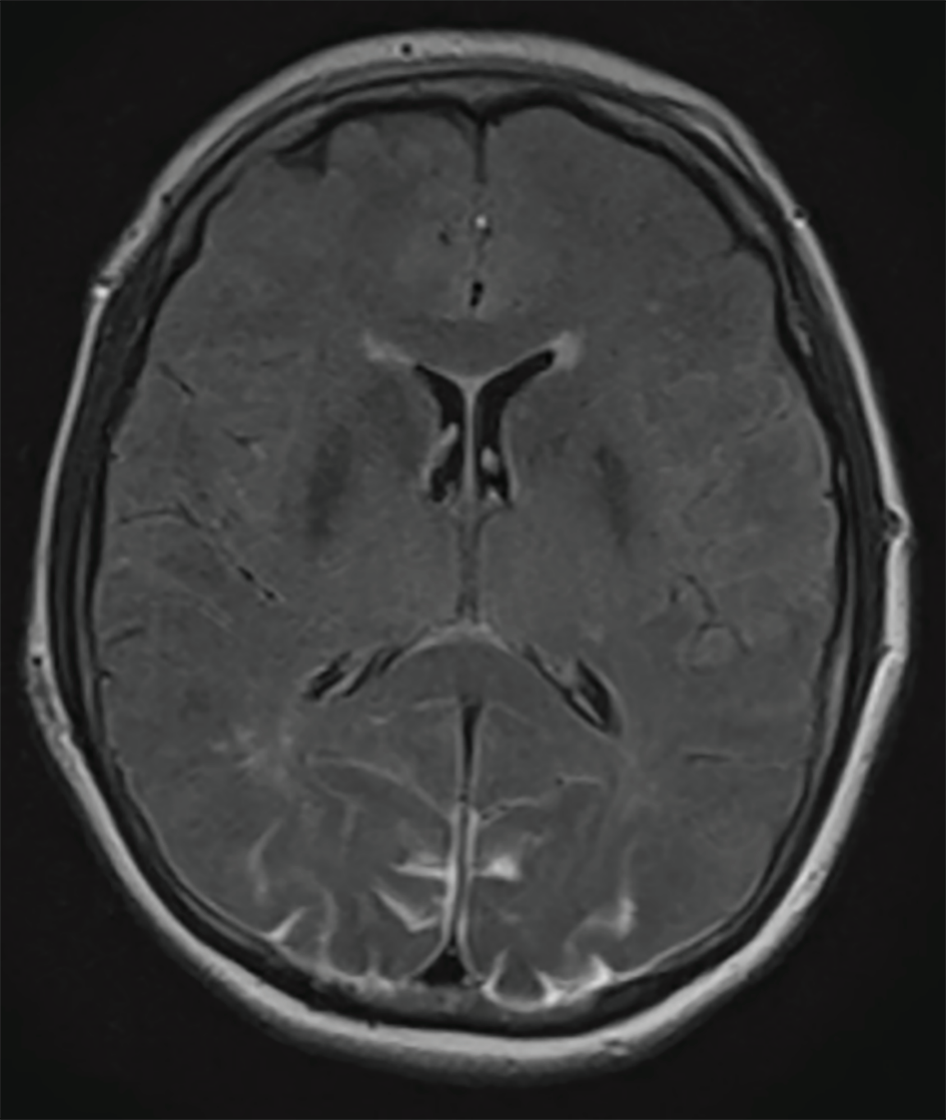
Axial FLAIR MRI demonstrating bilateral occipital lobe hyperintensity, suggestive of blood or edema. Because the images from other brain MRI sequences were normal, the patient was diagnosed with PRES.
